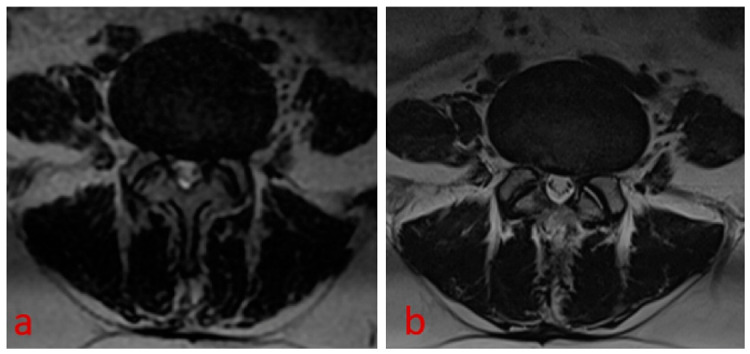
Representative axial lumbar MRI images of a patient undergoing percutaneous laser disc decompression. Legend: (a) Preoperative MRI showing lumbar disc protrusion associated with central canal narrowing and dural sac compression. (b) Three-month follow-up MRI after percutaneous laser disc decompression demonstrating partial reduction in disc protrusion and improved dural sac morphology. Radiological improvement should be interpreted cautiously because small changes in canal dimensions may be limited by measurement variability.
